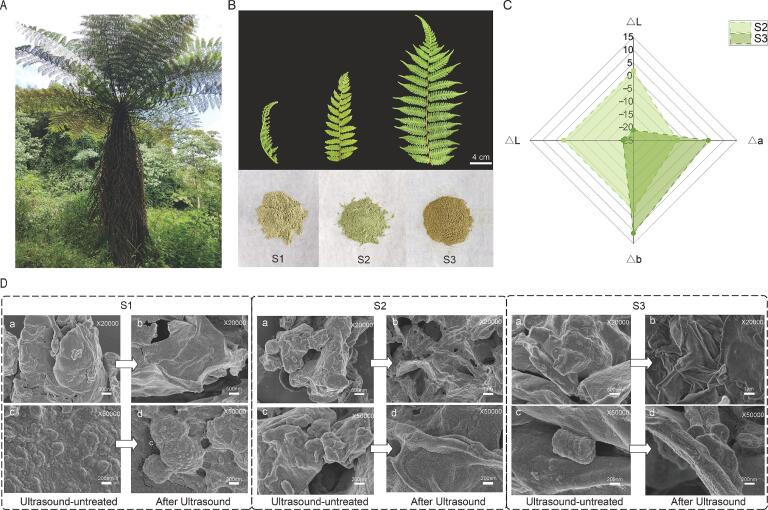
Morphology, colour traits, and SEM microstructure of *A. spinulosa* leaves at three maturity stages (S1-S3) before and after ultrasound treatment. (A) Representative plant image. (B) Representative fronds (top) and corresponding dried powders (bottom) from S1-S3. Scale bar = 4 cm. (C) Powder colour differences of S2 and S3 relative to S1, expressed as ΔL*, Δa*, and Δb*. (D) SEM images of S1-S3 powders before and after ultrasound treatment at × 20,000 and × 50,000 magnification.
